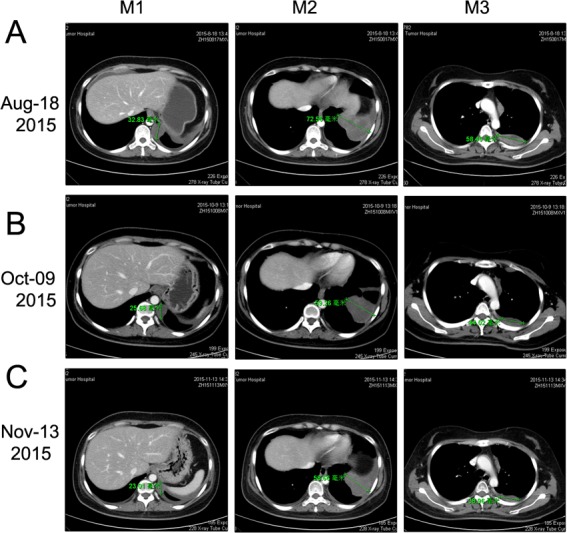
Chest CT scan documenting responses to Sunitinib A. After 4 weeks of treatment with Sunitinib (50 mg/day), CT image showing a SD (-14.12%, Aug 18, 2015). B. After about three months of treatment with Sunitinib, CT image showing a PR (-32.41%, Oct 09, 2015). C. After continuous treatment with Sunitinib for about four months, CT image showing a confirmed PR (-36.78%, Nov 13, 2015).
